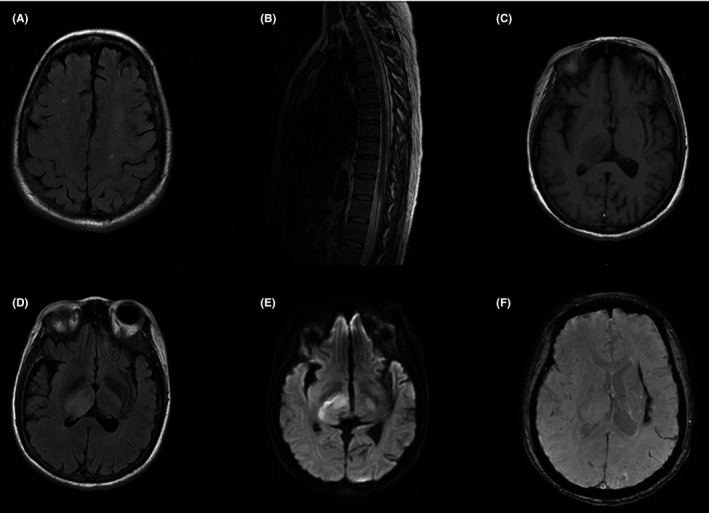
Magnetic resonance imaging (MRI) of the patient. (A, B) Previous MRI showed hyperintensive lesions in the centrum semiovale and thoracic spinal cord. (C‐E) MRI at this admission revealed right thalamic lesion, presenting with hypointensity on T1‐weighted images, hyperintensity on fluid‐attenuated inversion recovery sequences, and with restricted diffusion on diffusion‐weighted imaging. (F) Left basal ganglia hypointensity was observed on susceptibility weighted imaging, suggesting the deposition of hemosiderin after cerebral hemorrhage 3 years ago
